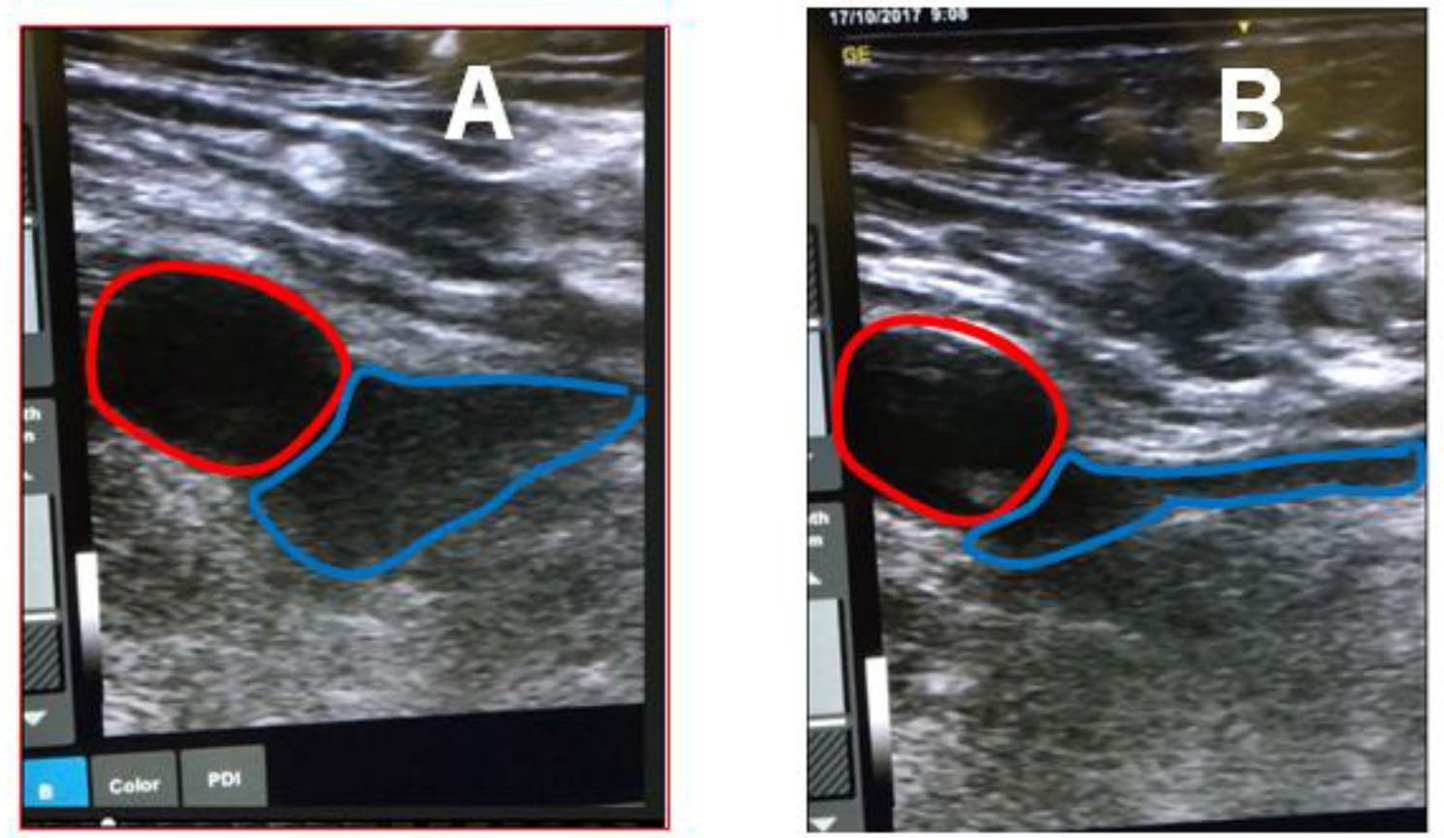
The use of ultrasound to differentiate between femoral arterial and venous circulation. A. Ultrasound image of right groin. Red structure represents femoral artery whereas blue structure represent femoral vein. B. Compression with ultrasound resulted into compressions of venous structure (blue) whereas arterial structure remained unchanged
